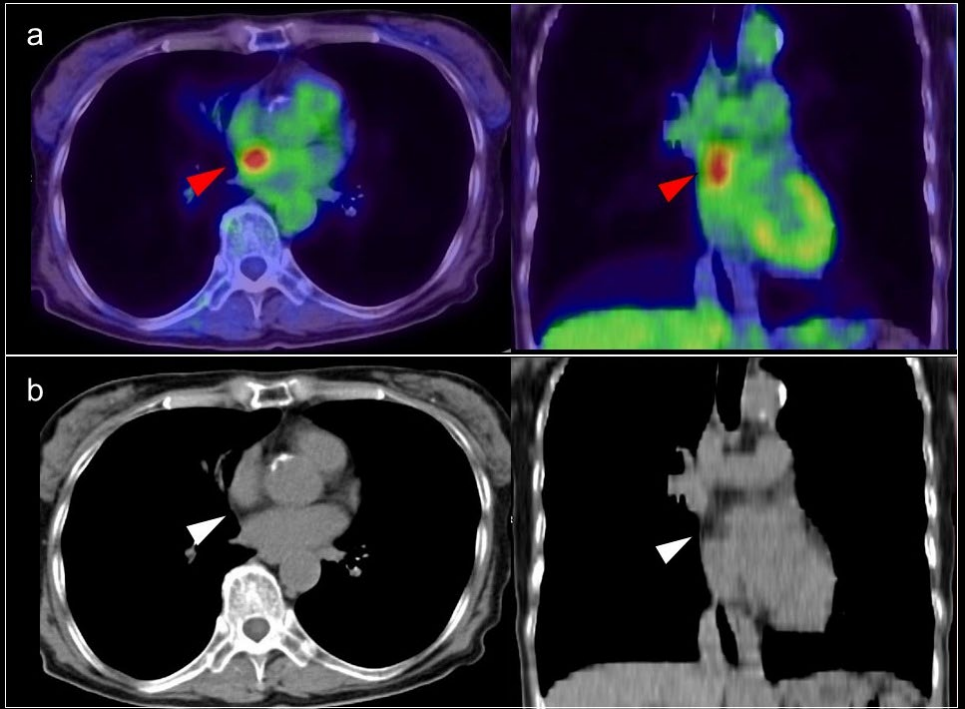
FDG uptake in lipomatous hypertrophy of the interatrial septum. Focal FDG uptake between left and right atrium (a red arrowheads), which corresponded to lipomatous hypertrophy of the interatrial septum (b white arrow heads)
